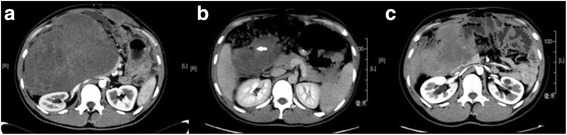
The progression of the intra-abdominal desmoid in patient 2. a CT scan before DOX/DTIC therapy showing a large mass in the abdomen and a desmoid tumor on the left abdominal wall. b CT scan of the same patient after DOX/DTIC therapy, showing tumor regression and perforation (as indicated by the *white arrow*, the gas was encapsulated by the tumor). c Follow-up CT scan in December 2015
